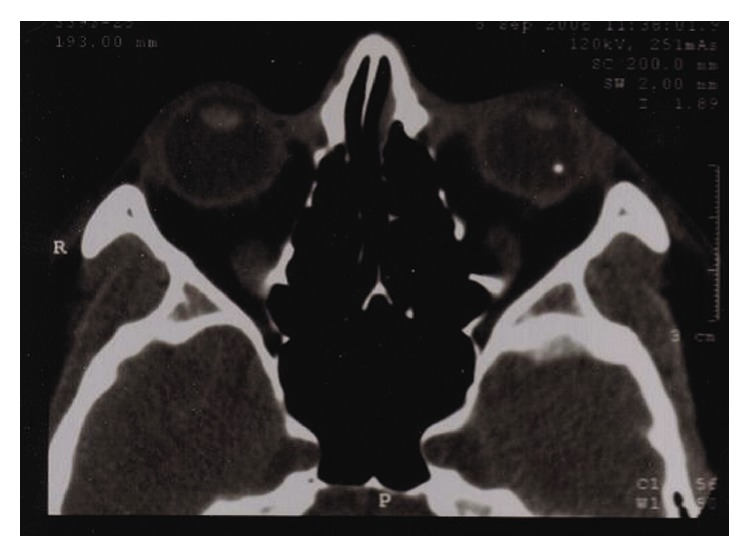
CT-scan depicting an intraocular foreign body in the left eye.
